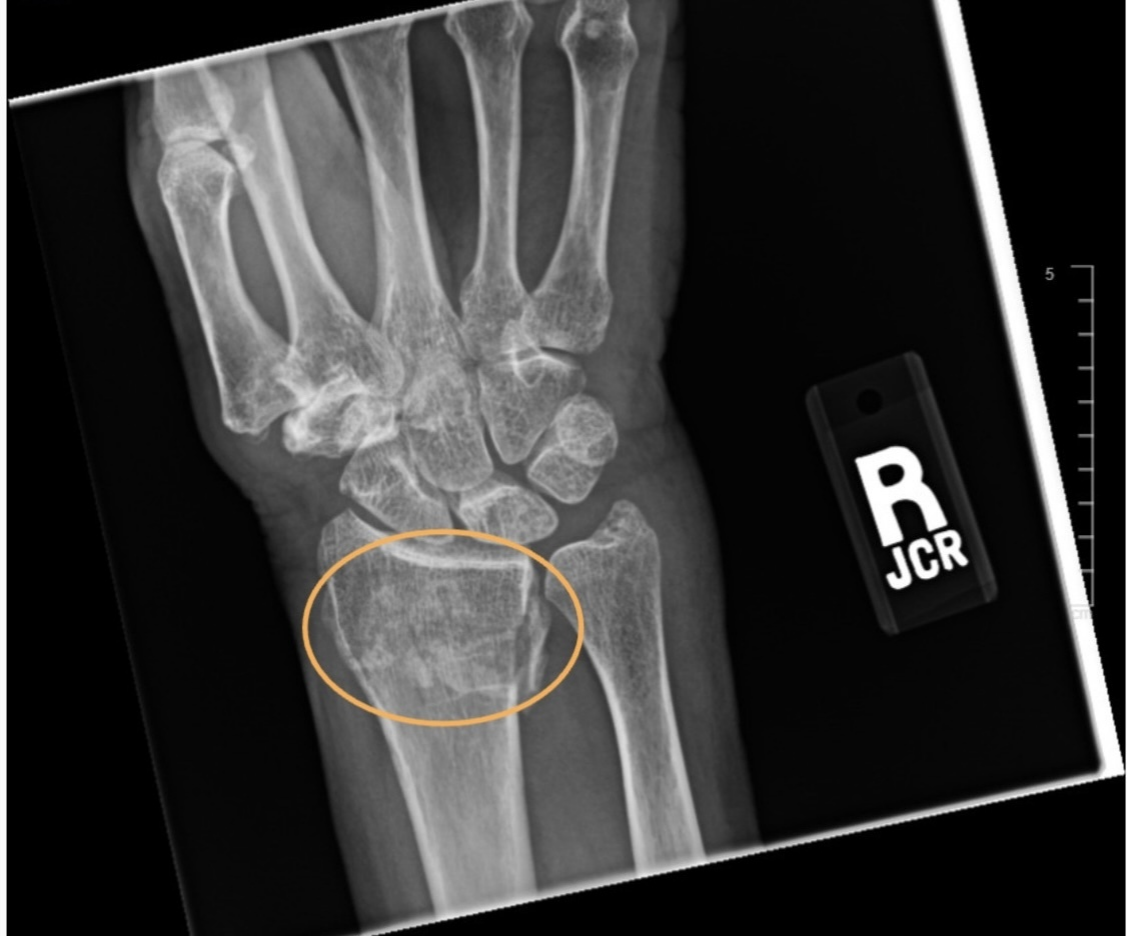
AP view wrist radiograph with the DRF circled in orange.
